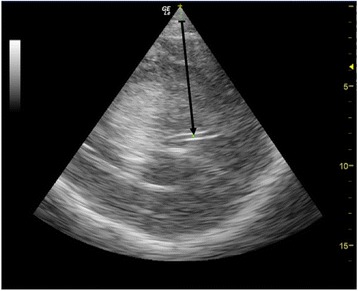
Example of visualisation of the third ventricle with brain sonography, the arrow pointing to the centre of the third ventricle.
